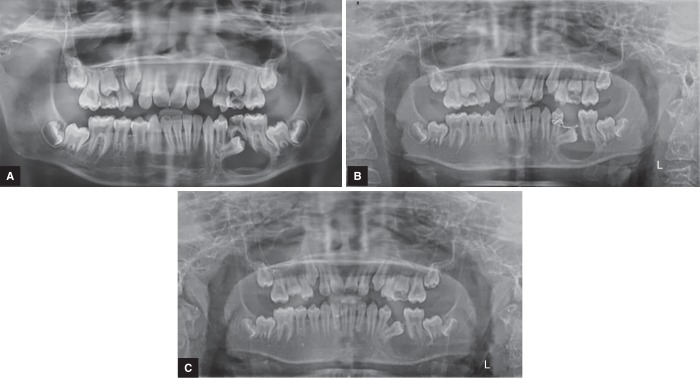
Panoramic radiographs: (A): Preoperative OPG; (B) Intraoperative OPG; (C) Postoperative OPG
